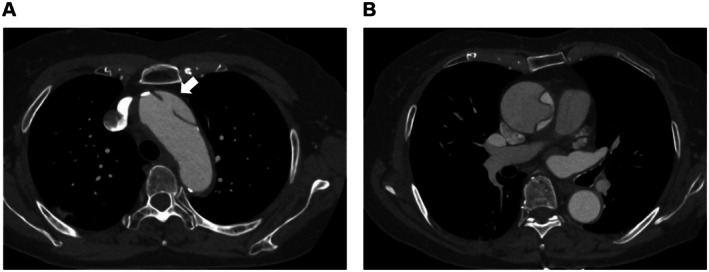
Preoperative contrast‐enhanced computed tomography. (A) The primary entry tear is located at the aortic arch (white arrow). (B) A patent false lumen is found in the ascending aorta, whereas the descending aorta remains intact.
